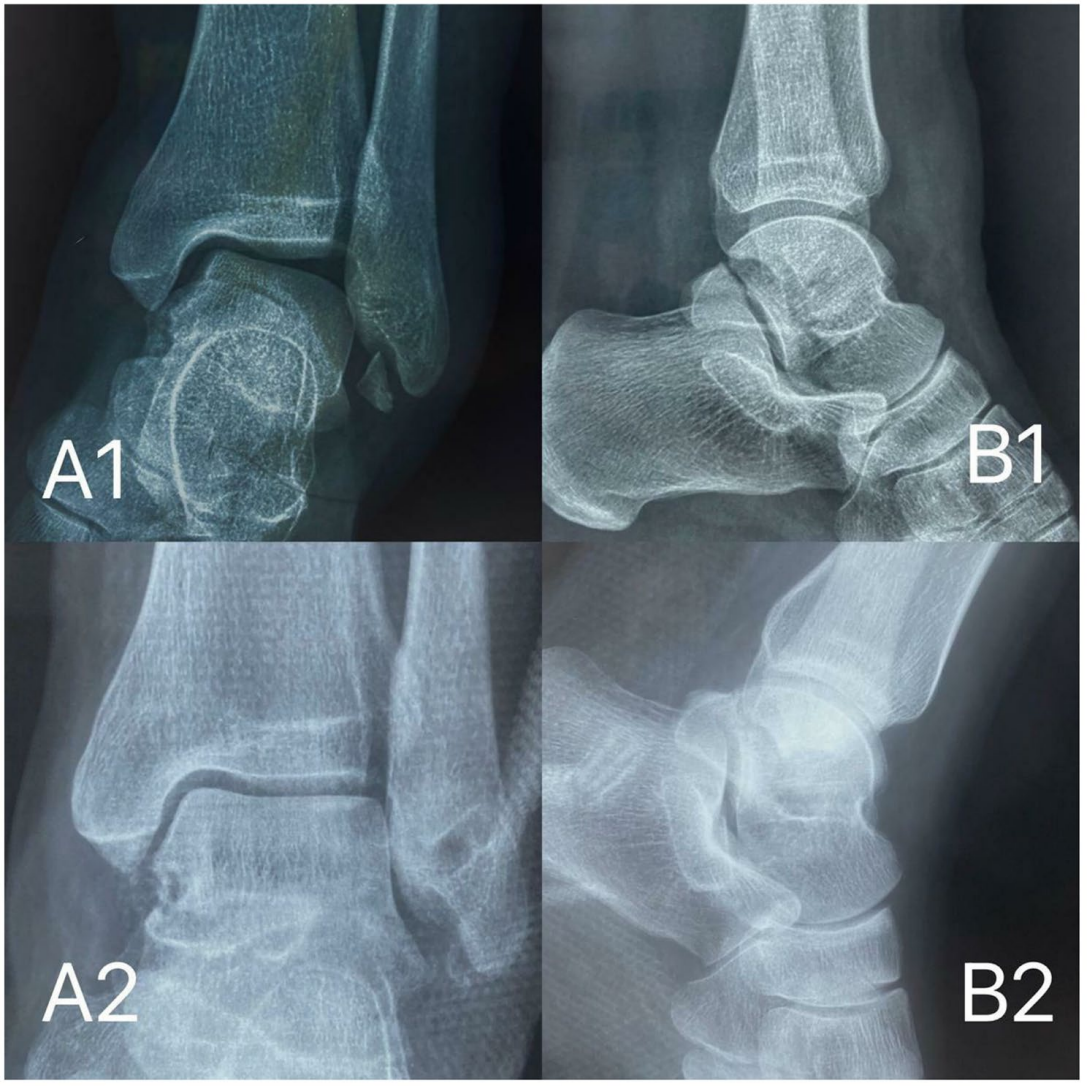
A 35-year-old female patient with right ankle sprain that lasted for 3 years was diagnosed with lateral instability of the right ankle and chronic lateral malleolar avulsion fracture. Preoperative stress level X-rays (A1, B1) and postoperative stress level X-rays (A2, B2)
